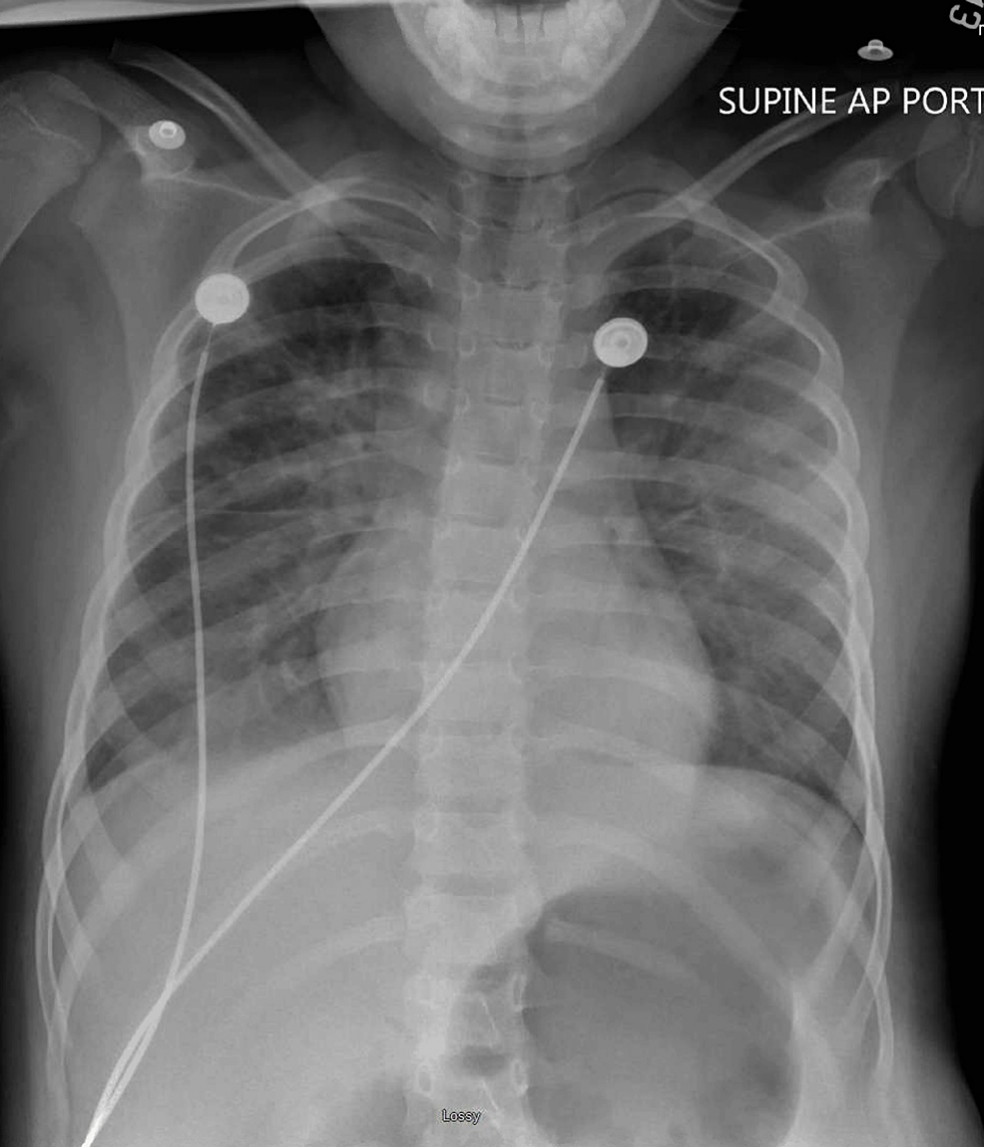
The patient's chest X-ray on day 2 of admission.
